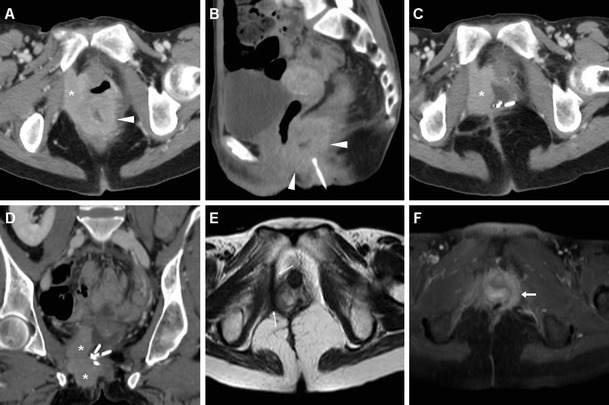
A 39-year-old woman with history of ulcerative colitis and perianal inflammation. Initial multiplanar MDCT (a, b) identified enhancing anal thickening (*arrowheads*) with right-sided vaginal infiltration and solid tissue (*) extending to reach the internal obturator muscle. After biopsy confirmation of SCAC and surgical debulking with colostomy, repeat MDCT (c, d) detected enlarging neoplastic residue (*). Shortly after chemo-radiotherapy, MRI (e) detected the formation of a thick hypointense fibrotic band in the site of the regressed tumour (*thin arrows*). MRI follow-up (e) identified appearance of a contralateral enhancing tissue band interpreted as suspicious for local recurrence (*arrow*). After negative clinical reassessment and PET findings, this post-treatment finding remained stable on further MRI studies (not shown)
